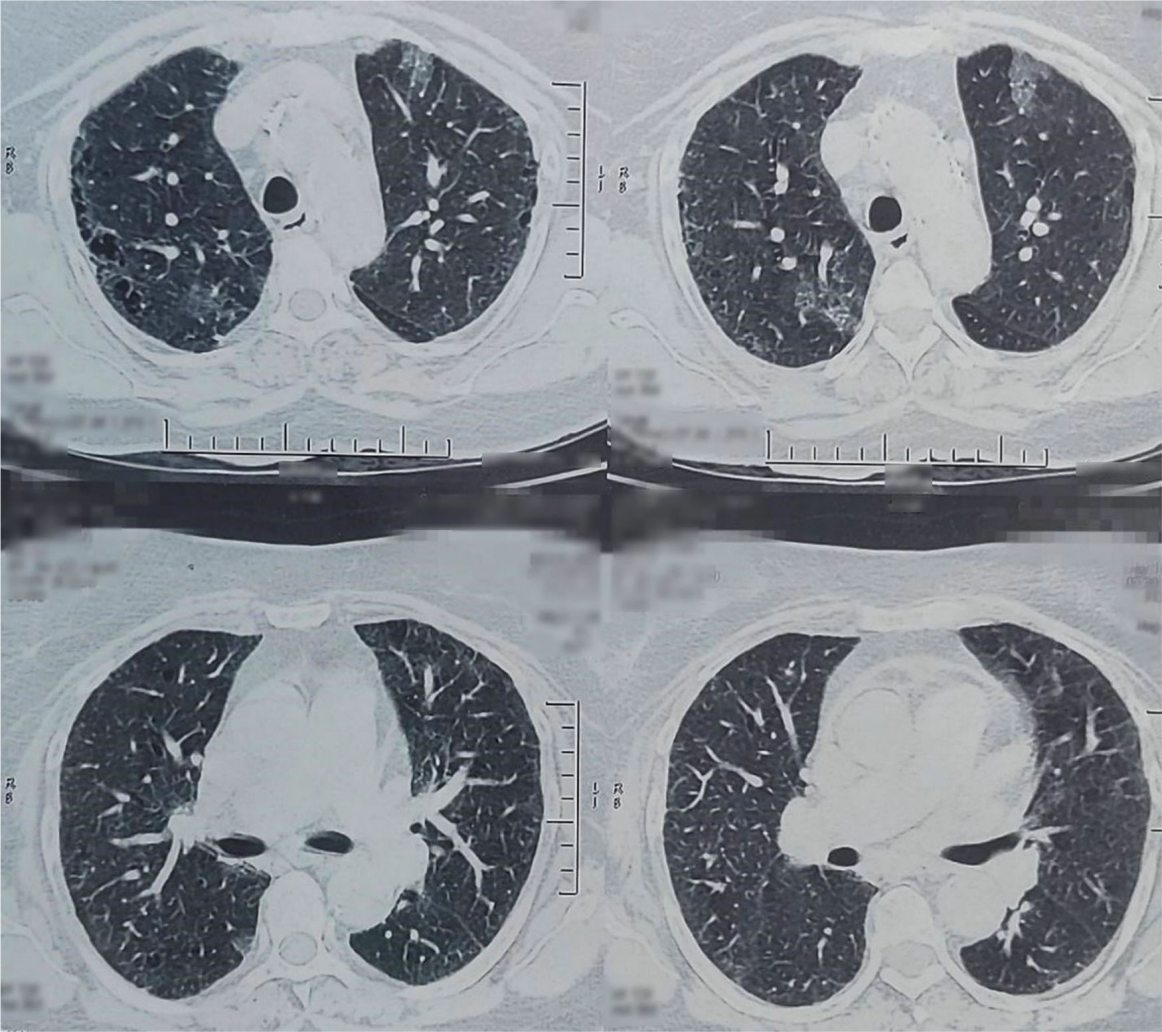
Axial section of computed tomography chest showing features of interstitial lung disease with chronic obstructive airway disease changes.
